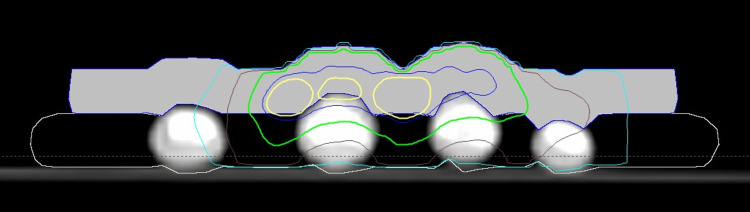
Example of electron dose distribution for knuckle pad treatment Using 6 MeV electrons prescribed to the 95% isodose line, knuckle pads of fingers two to five were treated using a 1 cm bolus between (beige outline) and a 1 cm bolus on top of the knuckle pads (solid light gray structure). The dose distribution shown here is at the level of the third finger knuckle pad (isodose lines: yellow, 95%; dark blue, 90%; green, 75%; brown, 50%; and light blue, 25%). Bolus outlines were manually created in the treatment planning system. The dose distribution for the second, fourth, and fifth finger knuckle pads is shown only partially, as knuckle pads in these fingers show in different CT planes CT: computed tomography
